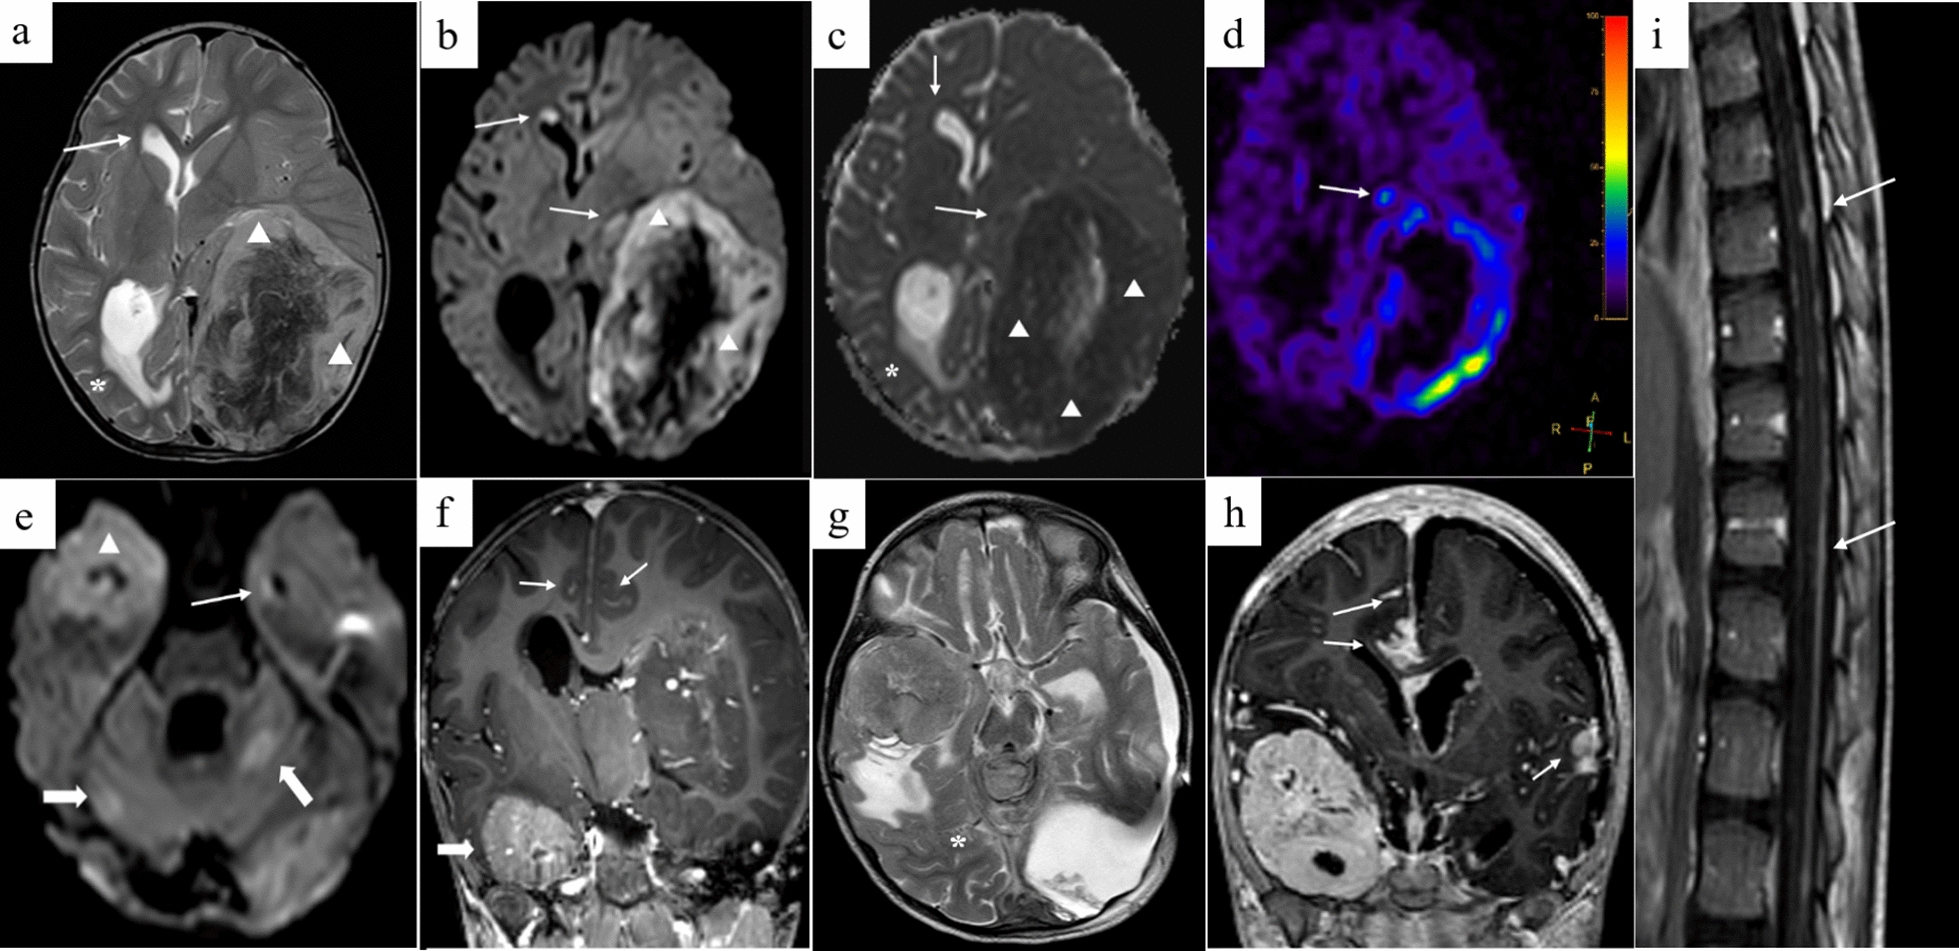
Radiological features. Pre-operative brain MRI a–f Images revealed a grossly well-marginated tumor mass lesion of approximately 8 × 9 cm located in the left parietal lobe. The lesion exerted severe mass effect on the ipsilateral lateral ventricle and adjacent brain parenchyma, resulting in contralateral active hydrocephalus (*in a and c) and midline shift. There was only mild peritumoral vasogenic edema. Signal intensity was strikingly heterogenous on all sequences suggesting the presence of core calcifications, necrosis and hemorrhage. The peripheral solid tumoral components displayed significant T2 prolongation (△ in a) and restricted diffusion on DW-images b, e and corresponding ADC map (△ in c), indicating high cellularity. ASL perfusion d demonstrated markedly increased CBF. Smaller size metastatic intraventricular (arrows in a–e), leptomeningeal (arrow in f) and parenchymal (bold arrows in e) lesions were present both supra-and infra-tentorially. The left parietal lobar lesion displayed faint, heterogenous contrast enhancement, whereas the right temporal lobe lesion enhanced avidly. The metastatic intraventricular deposits did not show any contrast uptake. Follow-up brain (g, h) and spinal cord (i) MRI: post contrast coronal T1-weighted (h) and sagittal T1-weighted (i) images of the brain and spinal cord, four weeks after diagnosis, demonstrated marked interval tumoral increase in size and progression of subependymal and leptomeningeal dissemination (arrows). Surrounding vasogenic edema was also increased (*in g). DW: diffusion-weighted; ADC, apparent diffusion coefficient; ASL, arterial spin labeling; CBF, cerebral blood flow
